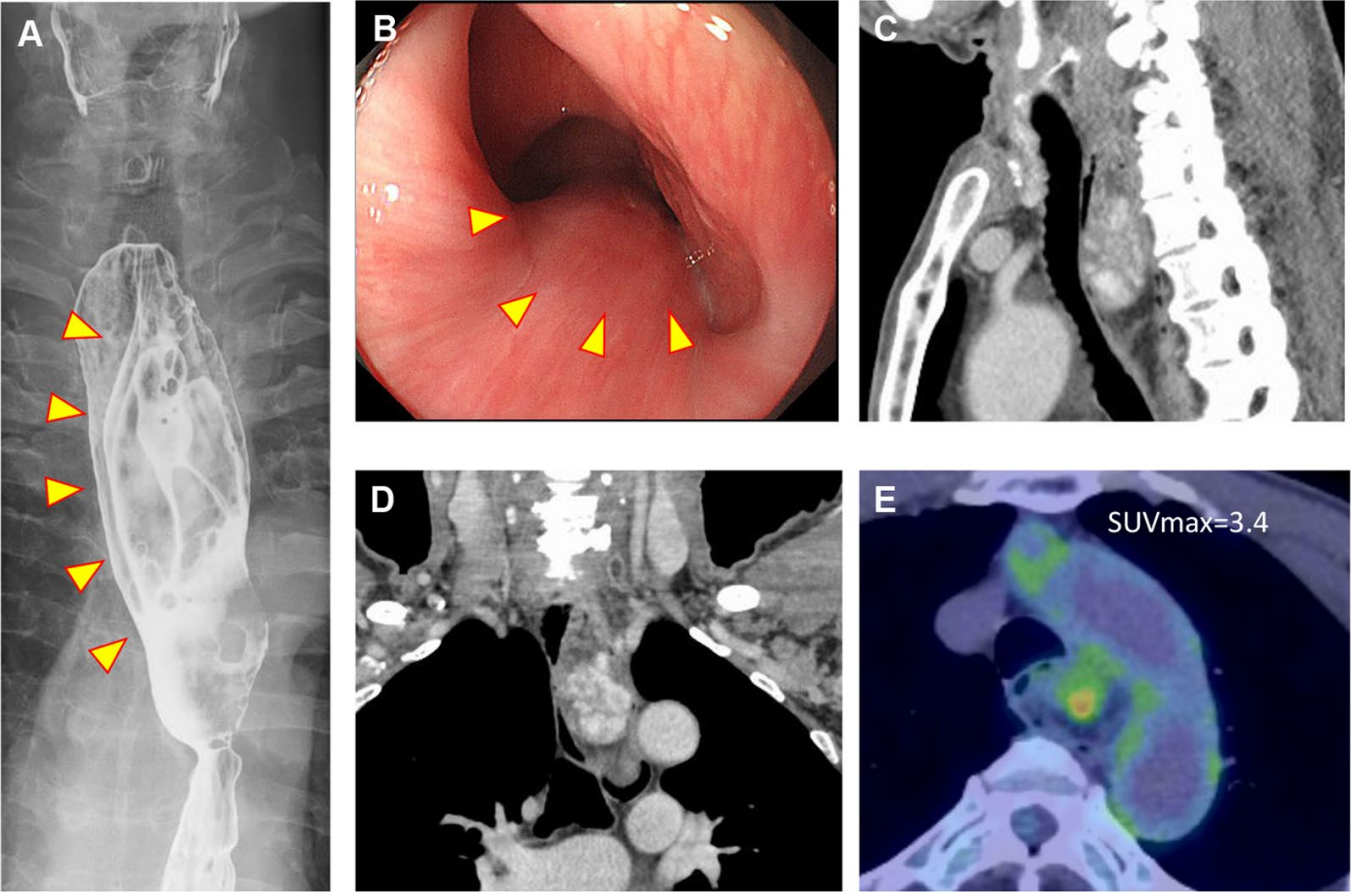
Preoperative X-ray fluoroscopy, upper gastrointestinal endoscopy, and PET–CT. A The tumor extends from the cervical esophagus to the upper thoracic esophagus. B Tumor stalk arose from the esophageal entrance. C, D The tumor was located from neck to the middle intrathoracic esophagus. The inside was a mixture of rich components with a potentiating effect and fatty components. E A low level of FDG accumulated (SUV max = 3.4) at the part of the enrichment of the tumor. There were no findings suggesting the invasion of tumor cells to neighboring organs
